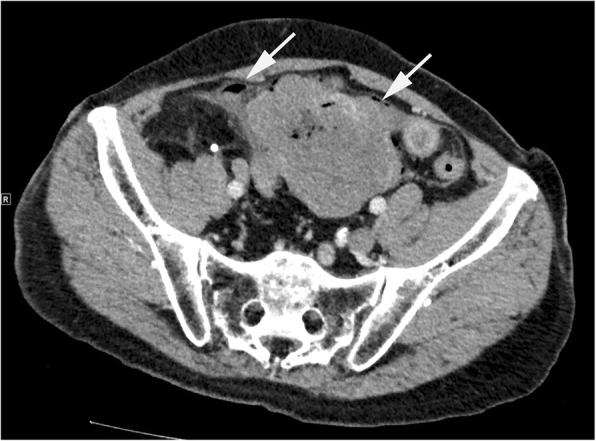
Emergent computed tomography images on the day of the operation. Arrows represent free air close to the tumor
